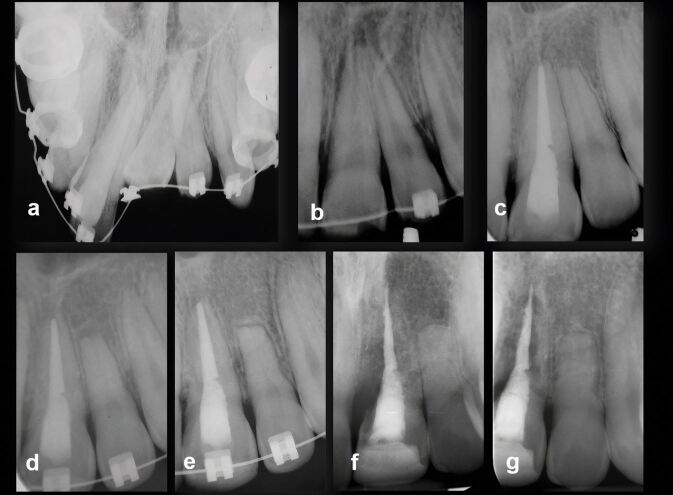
Male patient (13 years old) undergoing a course of OTM suffered a golf club injury resulting in tooth 11 luxated superolabially, 21 intruded with only incisal edge visible and uncomplicated crown fracture, and 22 intruded approximately half the crown height. a) Pre-operative occlusal radiograph. b) March 1997, teeth repositioned and splinted. c) April 1998, 13-month review showing completed root canal treatment of tooth 21 and remodelled tooth 22 undergoing PCC. d) 23-month review, OTM commenced, tooth 22 showing evidence of transient apical breakdown, orthodontist requested to pause OTM. e) 31-month review showing continued PCC of 22 and apical root remodelling. f, g) 22.5-year review showing advanced replacement resorption of 21, and PCC of 22 with normal apical tissues (panels f and g courtesy of Dr Vijay John)
